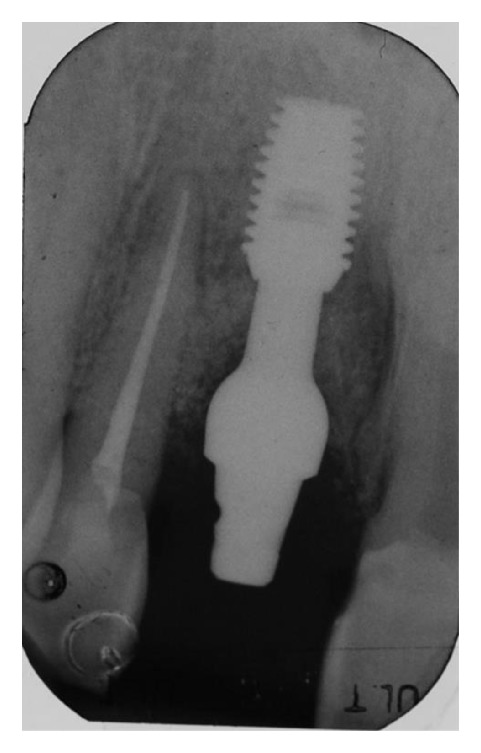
Radiograph showing the long abutment post, chosen to respect the bone and mucosal tunnel.
